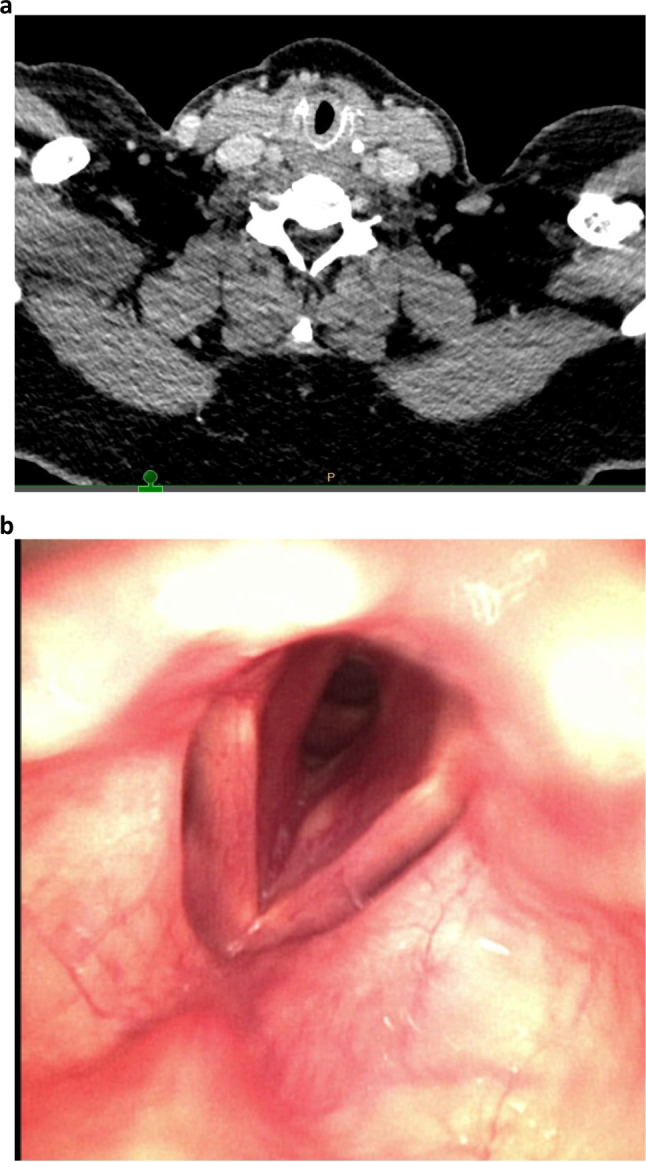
Computed tomography (CT) scan (a) and flexible endoscopy (b) in a 54-year-old female patient with a nine-month history of exertional shortness of breath and stridor. a Circumferential scar tissue directly under the true vocal cords. b Subglottic stenosis with obstruction of approximately 50%
